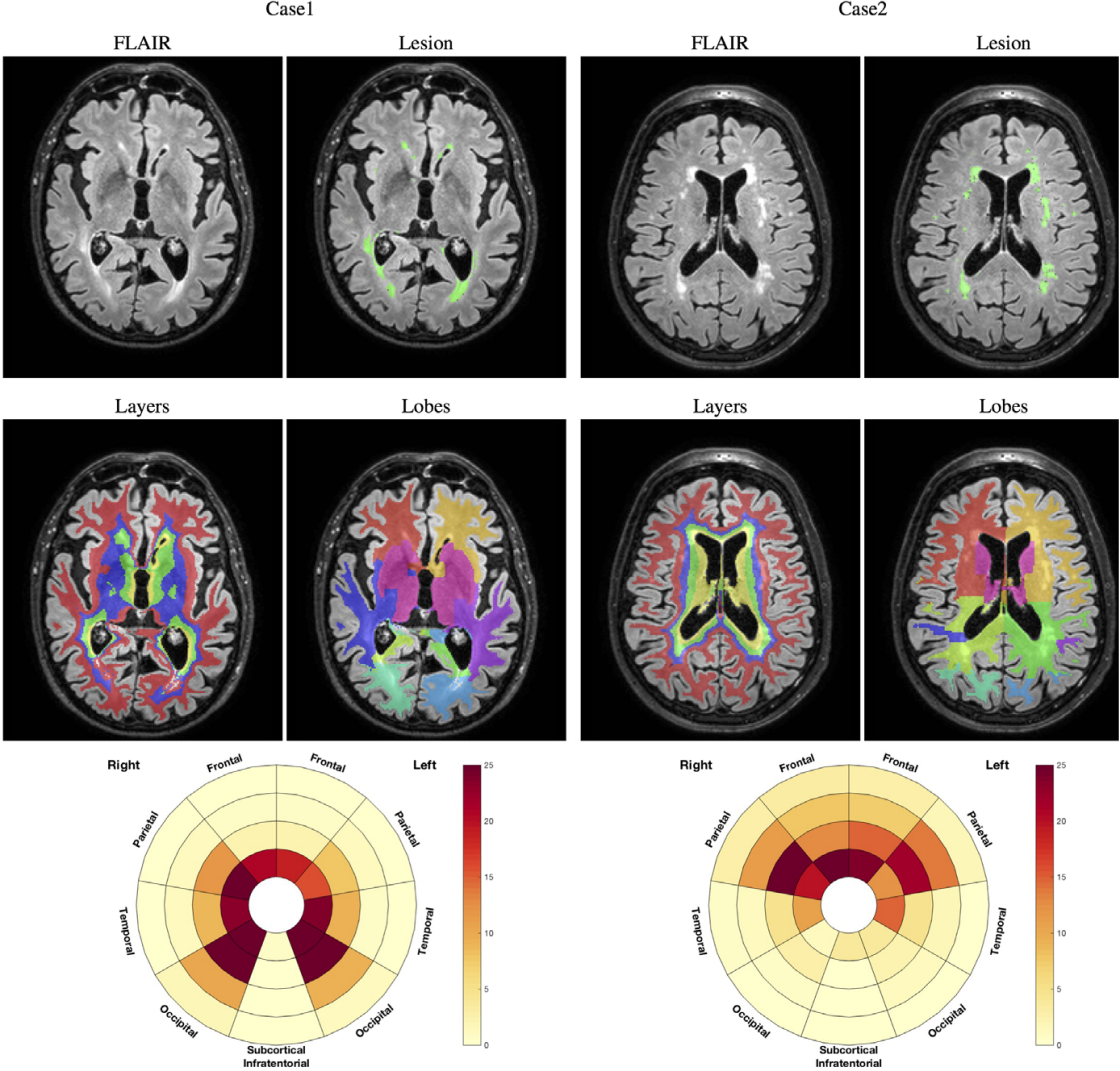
Example of 2 cases with original FLAIR image and lesion segmentation (first row), layers and lobar separation (second row), and resulting bullseyes plot. The color indicates the percentage of a given region to be occupied by WMH. The angular segment represents the different lobes while concentric layers indicate distance layers from the ventricular surface, distance increasing with the radius. Example of 2 cases with original FLAIR image and lesion segmentation (first row), layers and lobar separation (second row), and resulting bullseyes plot. The color indicates the percentage of a given region to be occupied by WMH. The angular segment represents the different lobes while concentric layers indicate distance layers from the ventricular surface, with distance increasing with the radius. FLAIR indicates Fluid Attenuated Inversion Recovery; WMH, white matter hyperintensities.
